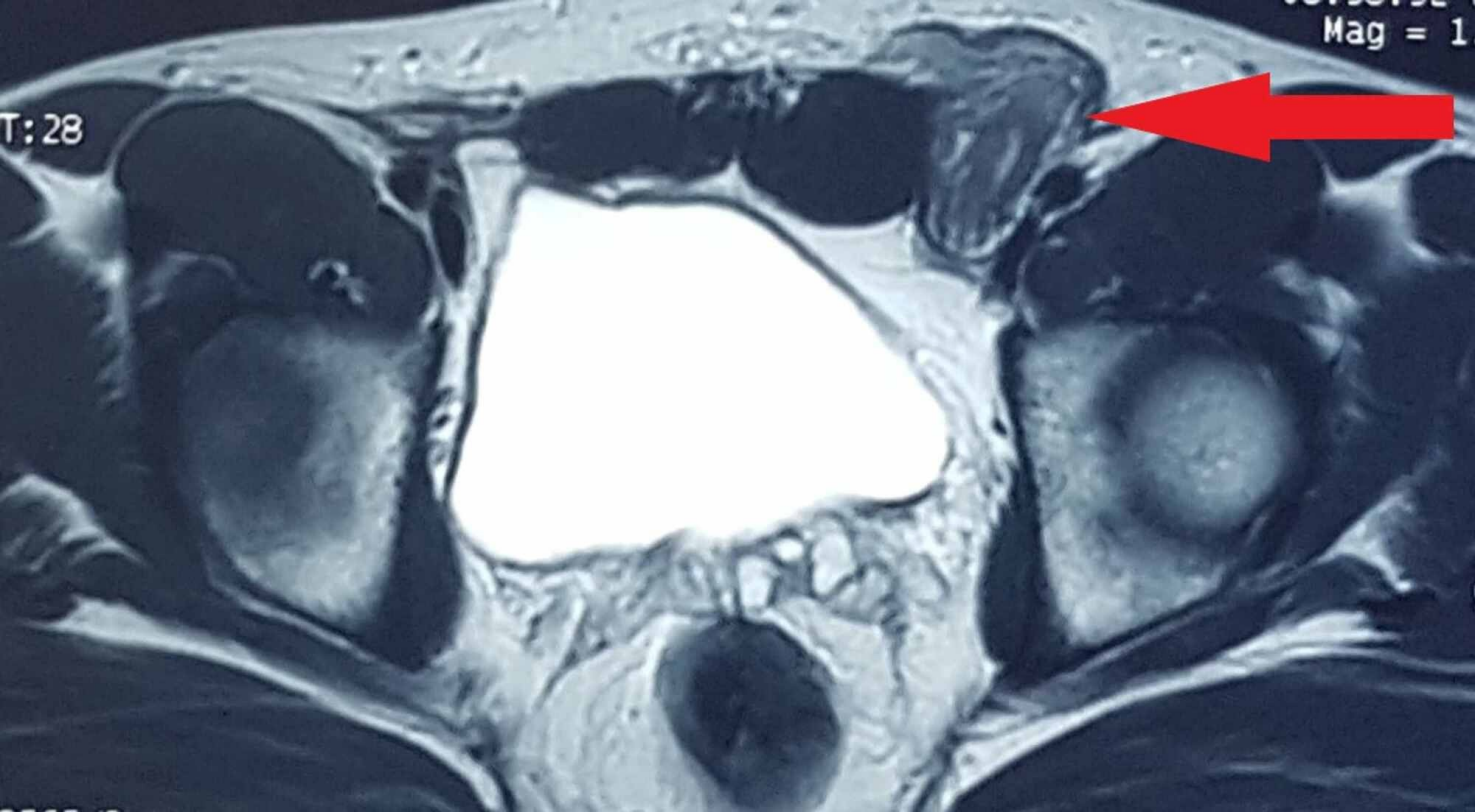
MRI T2-weighted images showing thickened left spermatic cord.
